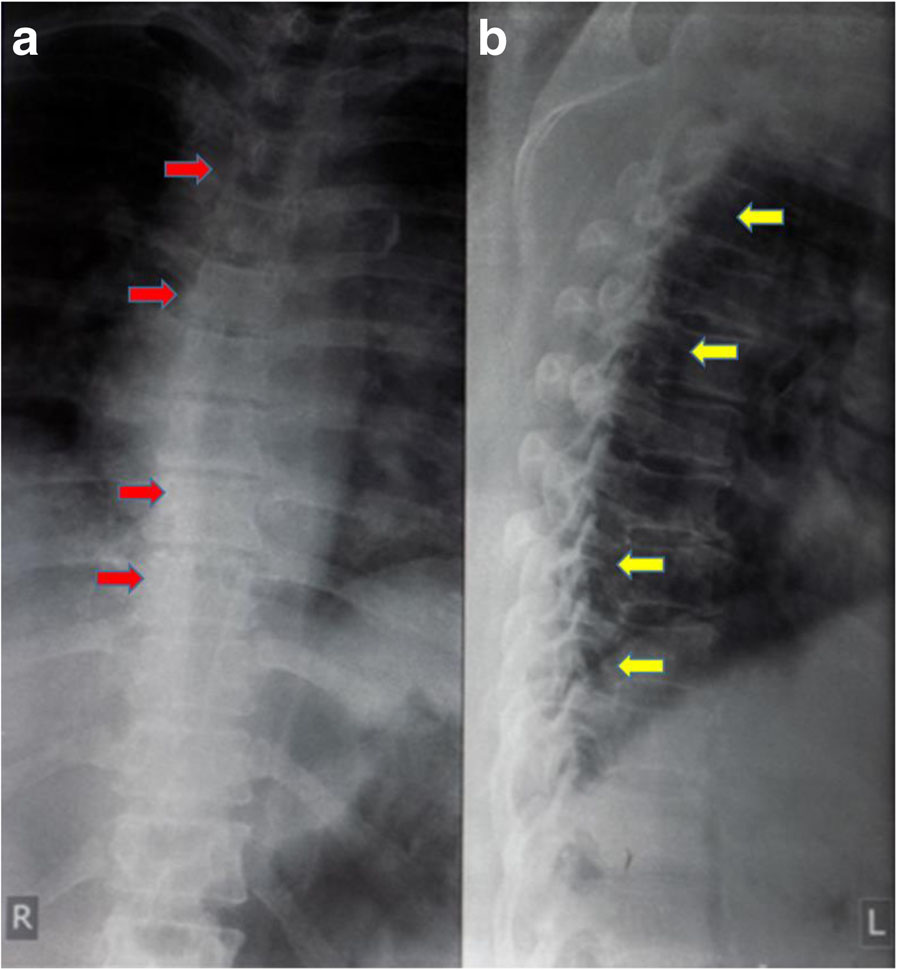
Plain radiograph of thoracic region, anteroposterior and lateral views. a Anteroposterior view shows spinal deformity and mass deposition process around the thoracic vertebrae (*red arrows*). b Lateral view shows degenerative process with mass deposition at some level of the spinal canal (*yellow arrows*)
